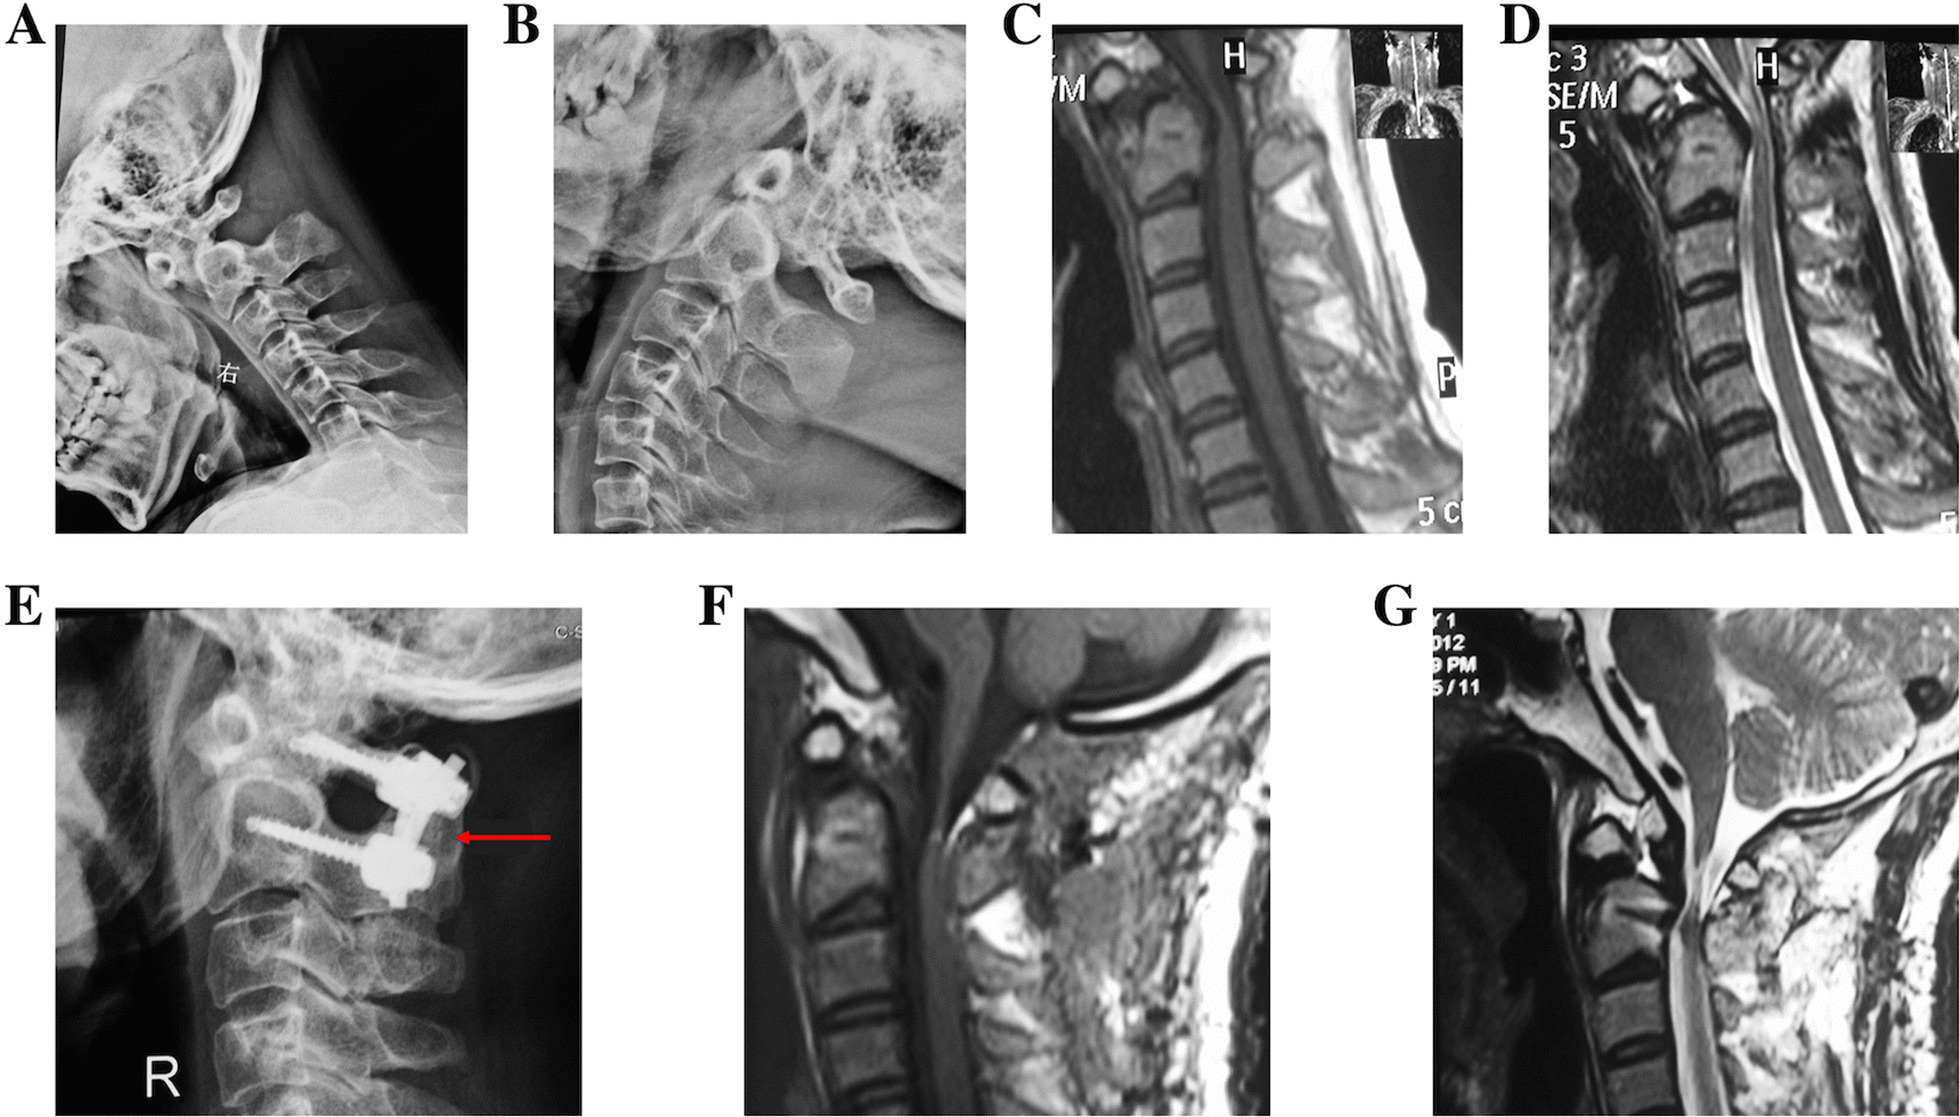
A 45-year-old male patient was admitted for numbness of limbs and walking instability for 2 years. Preoperative dynamic radiographs (a, b) showed successful reduction of the atlantoaxial dislocation in a hyperextended position, so it was classified as type I. Preoperative T1-weighted MRI showed atrophy of the spinal cord (c), and T2-weighted MRI showed high signal intensity of the spinal cord (d). Following posterior fixation and fusion, satisfactory reduction and internal fixation were achieved (e, red arrow). Postoperative MRI showed that spinal cord compression was relieved (f, g), and the patients had further neurological deterioration within 6 months after operation
